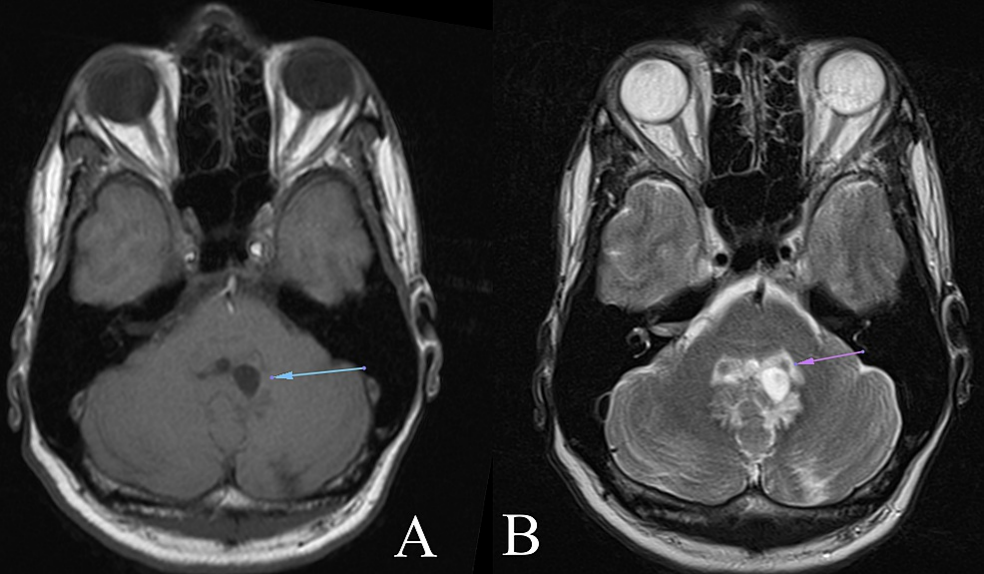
Axial T1 and T2-weighted MRI showing dilated foramina of Luschka (image A, arrow) and engorged choroid plexus (image B, arrow)
